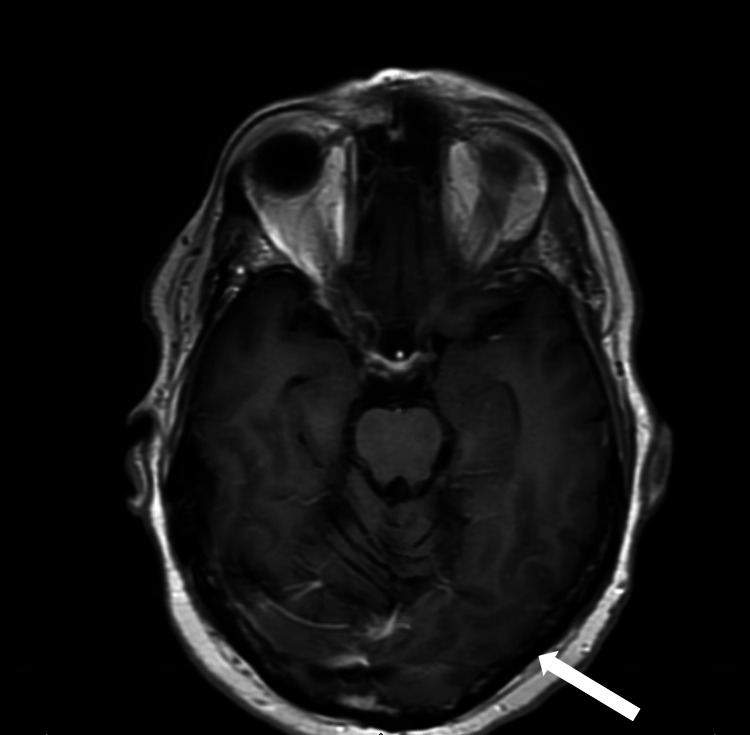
Repeat brain MRI after six months showing interval improvement of the contrast enhancement in the left occipital lobe (white arrow)
